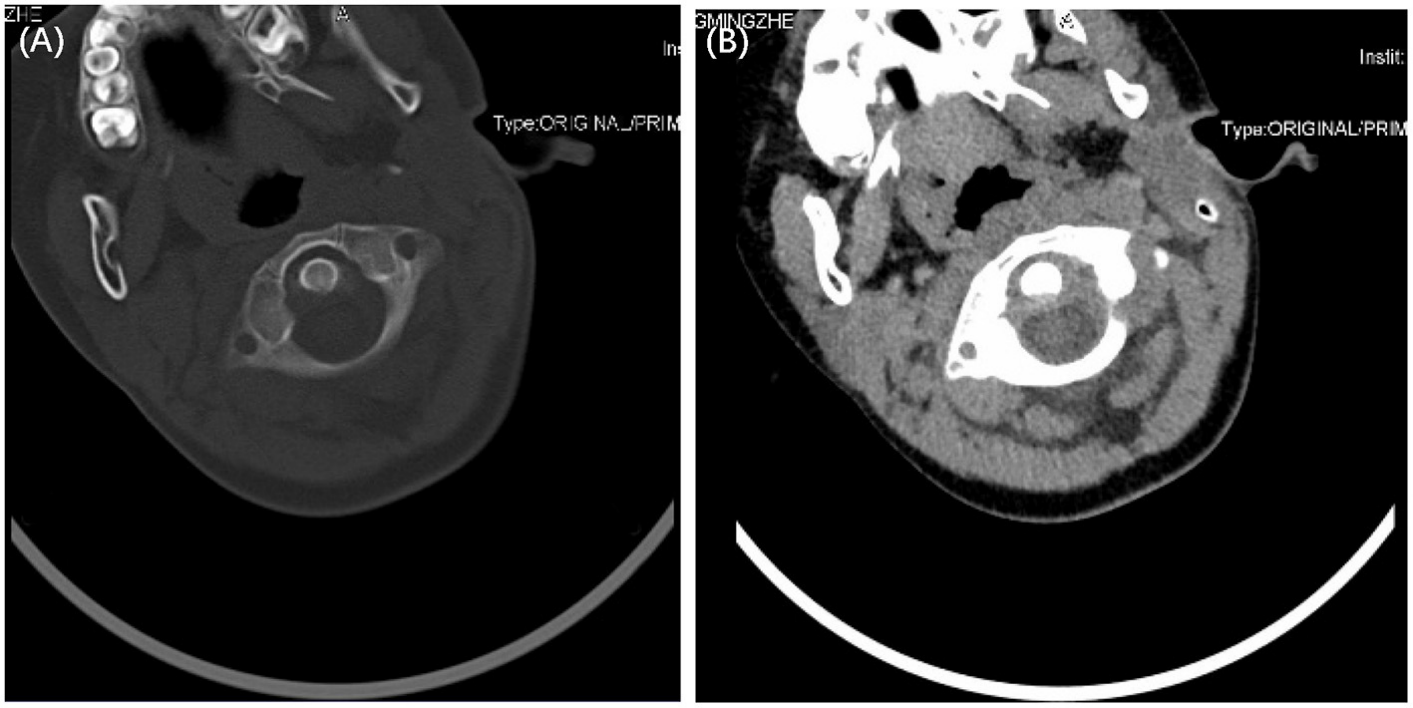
A computed tomography scanning showed Type I atlantoaxial joint subluxation (A,B).
